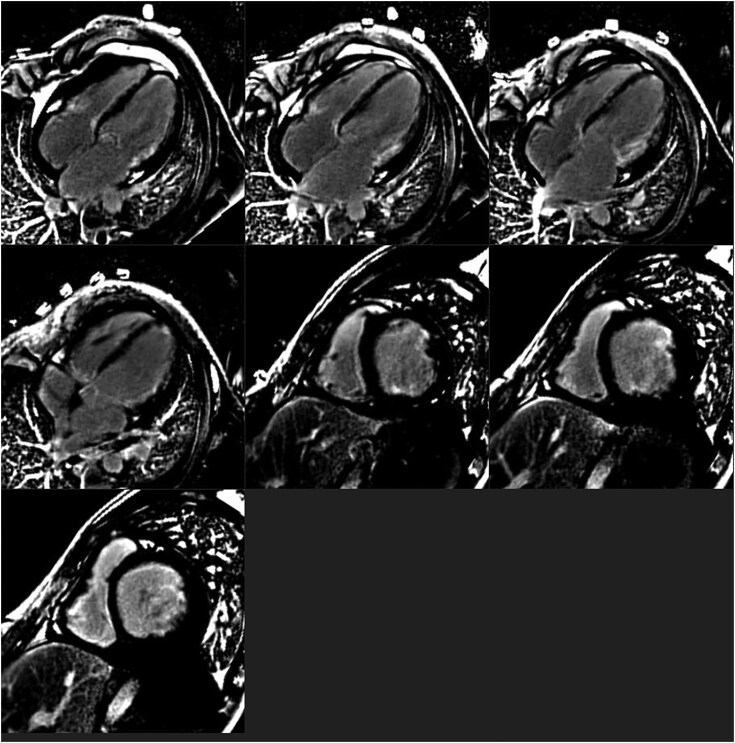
Cardiac MRI shows multifocal, patchy, subendocardial LGE, patchy subepicardial LGE in mid- to apical cavity inferior segments.
